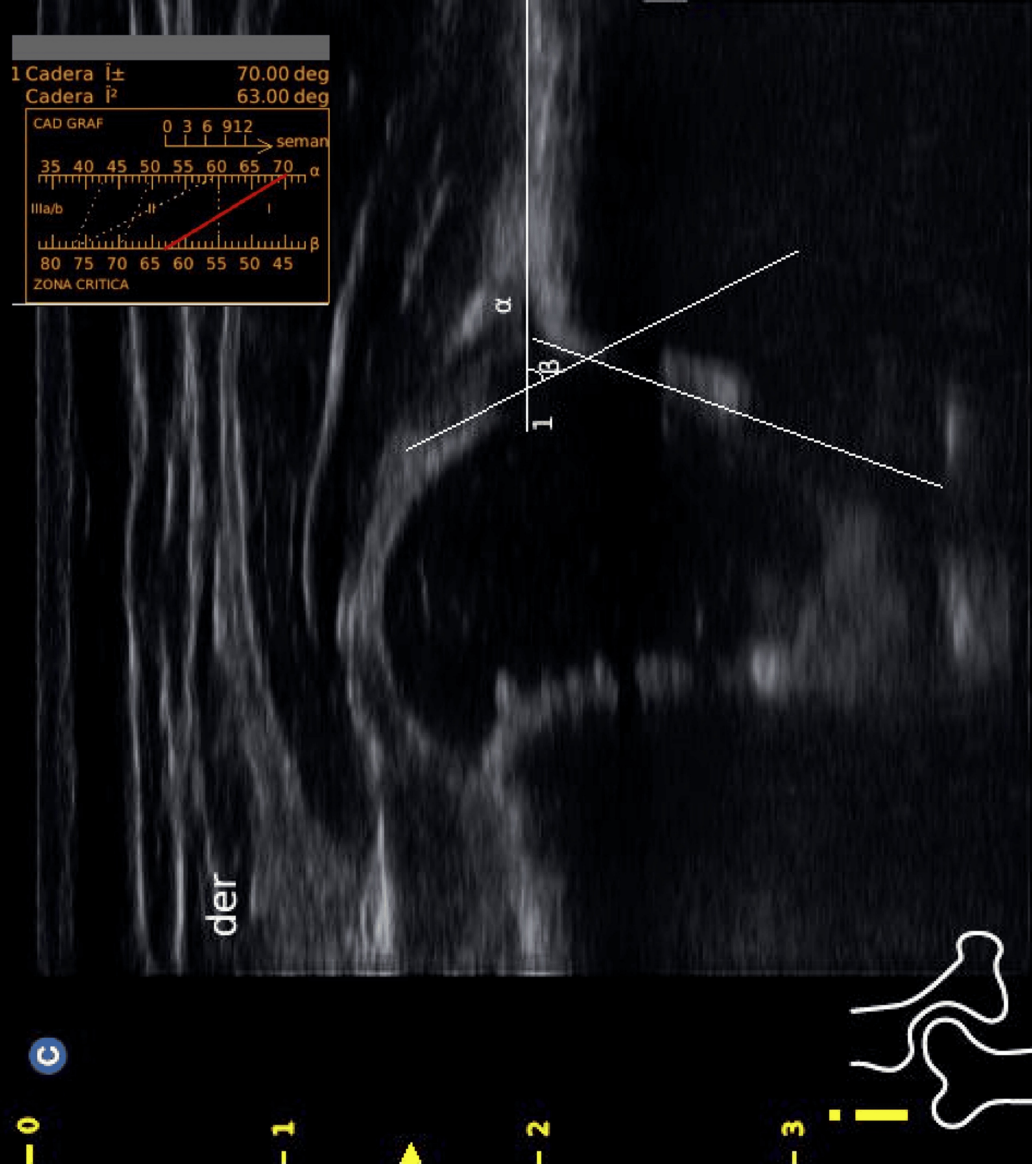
Ultrasonography of the hip: measurement of alpha and beta angles
